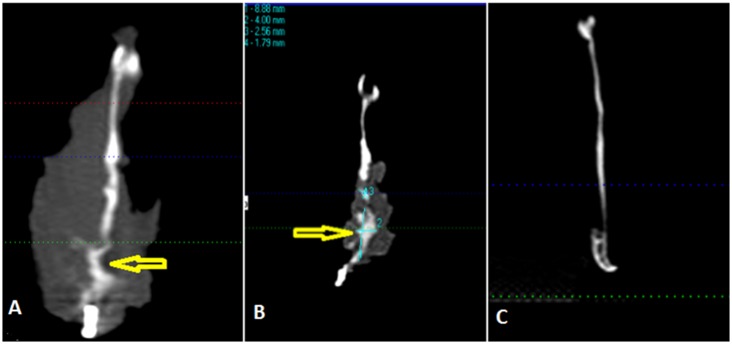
Coronal radiographic scan through the ramus of the mandible; the thickness of the slice is 1.6 mm. A, shows the bone regeneration area (yellow arrow). B, shows linear measurement of the newly formed bone. C shows coronal section of the bone of the contra-lateral side.
